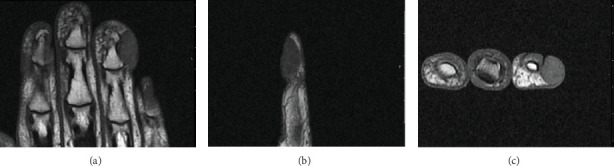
(a–c) MRI prior to marginal excisional biopsy.
